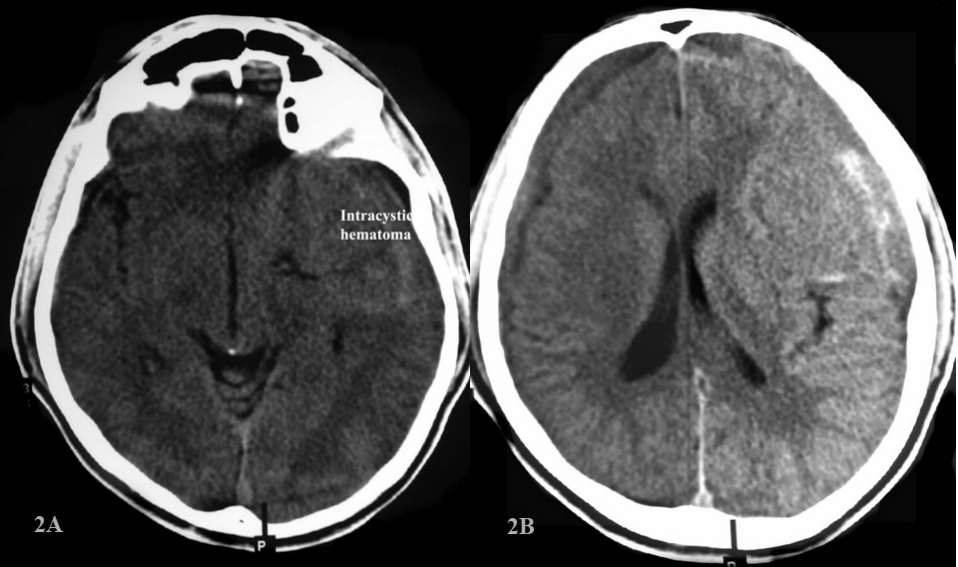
Computed tomographic scan of the head without contrast 3 weeks after the trauma. Figure 2A shows an intracystic hematoma. Figure 2B shows a bilateral subdural hematoma of mixed density.
